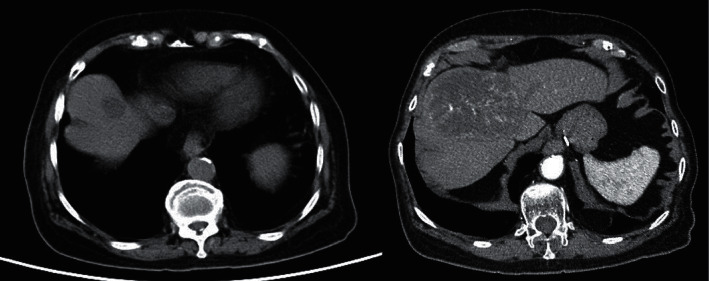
(a) Non-contrast CT abdomen of Patient B in October 2017 showing reduction of liver metastasis 8 months after mitotane (compared with Figure 7). (b) Contrast CT abdomen of Patient B in April 2018 showing the progression of liver metastasis 14 months after mitotane.
